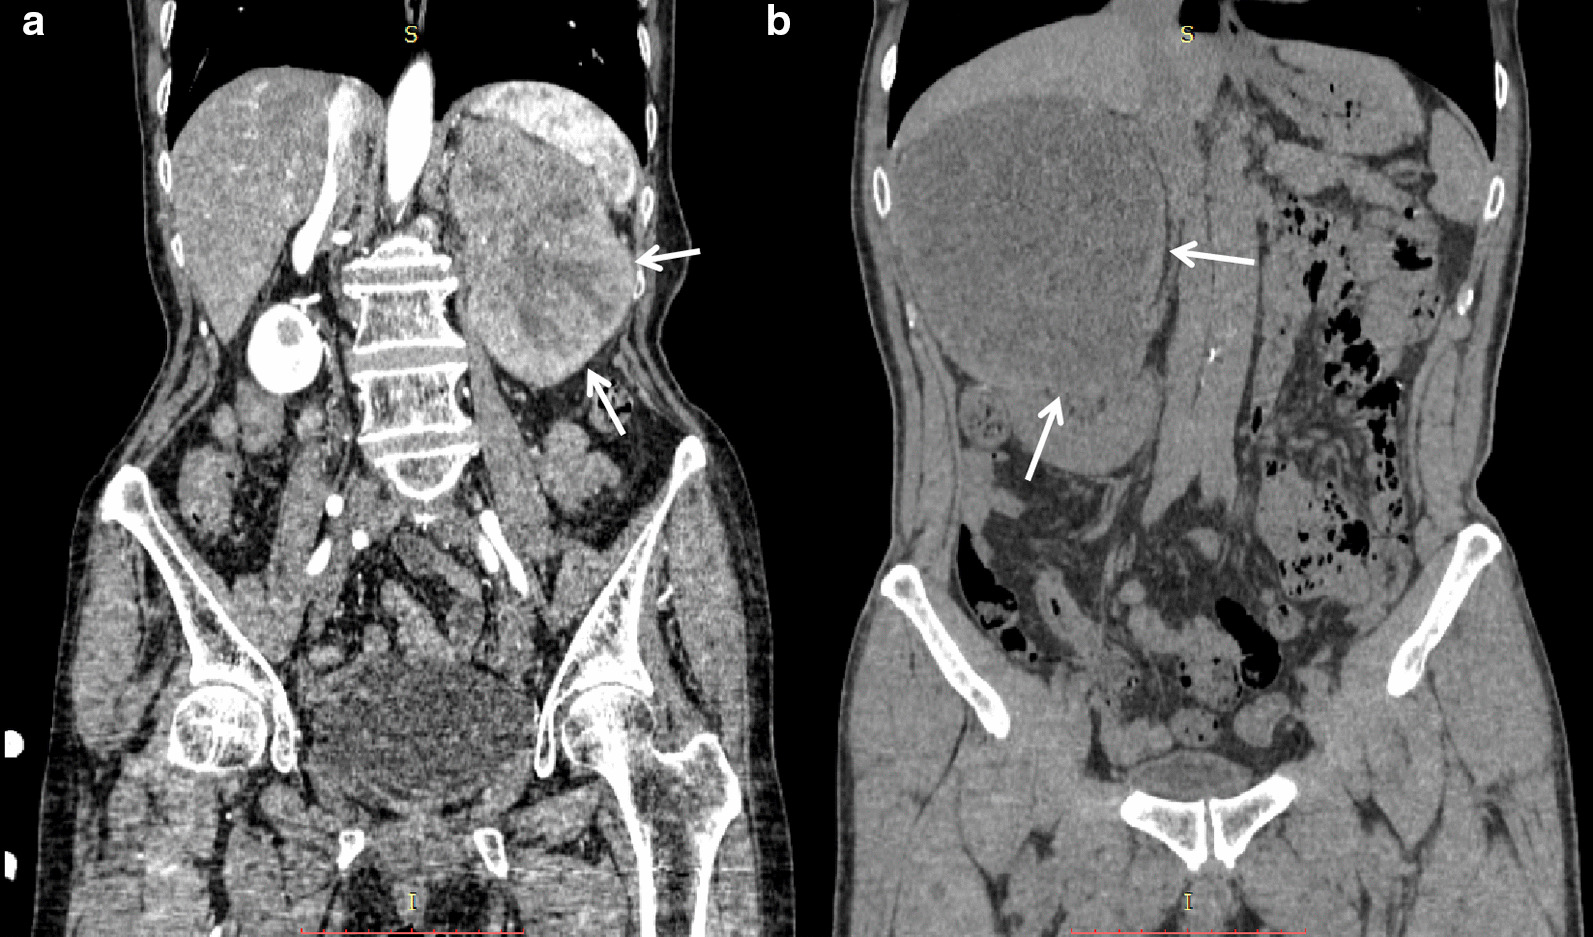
a Case 8: coronal enhanced CT image shows a left kidney collecting ductal carcinoma (CDC). The tumor involves the renal cortex, medulla, and renal pelvis. The boundary is unclear (arrows). b Case 12: coronal unenhanced CT image shows a right kidney mass-type CDC. The tumor compresses the surrounding renal cortex and medulla. The boundary is obvious, and is considered the compressed renal cortex (arrows)
